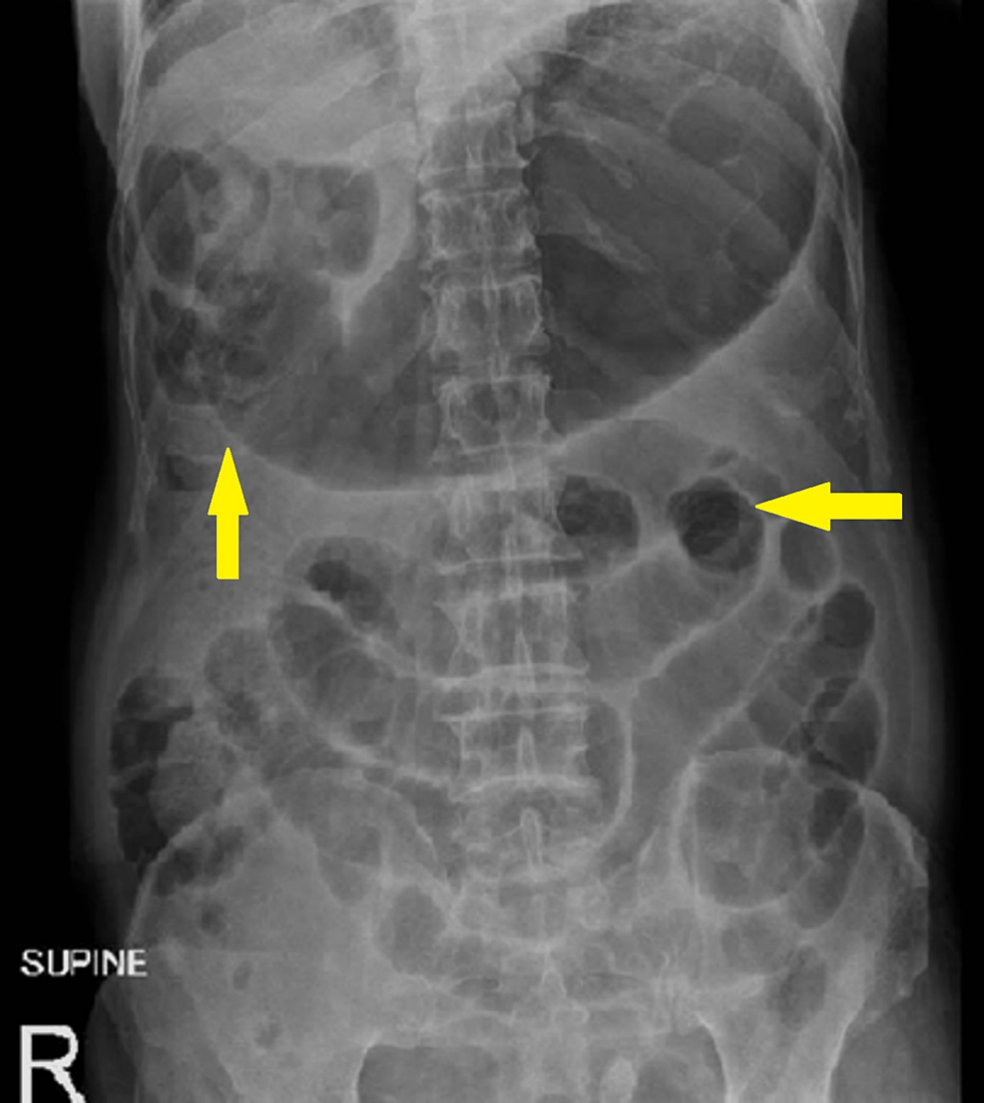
Abdominal supine x-ray demonstrating significant gastric distension and multiple dilated small bowel loops.
